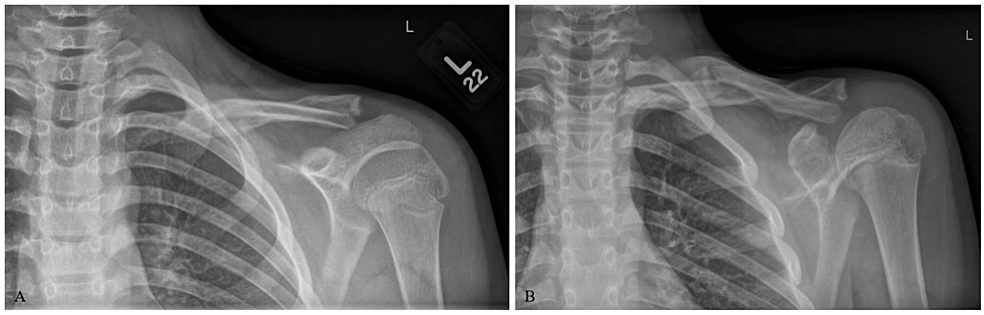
Six-week post-injury radiographs, upright anteroposterior and Zanca views (A-B), demonstrating interval healing and callus formation at the fracture sites.
